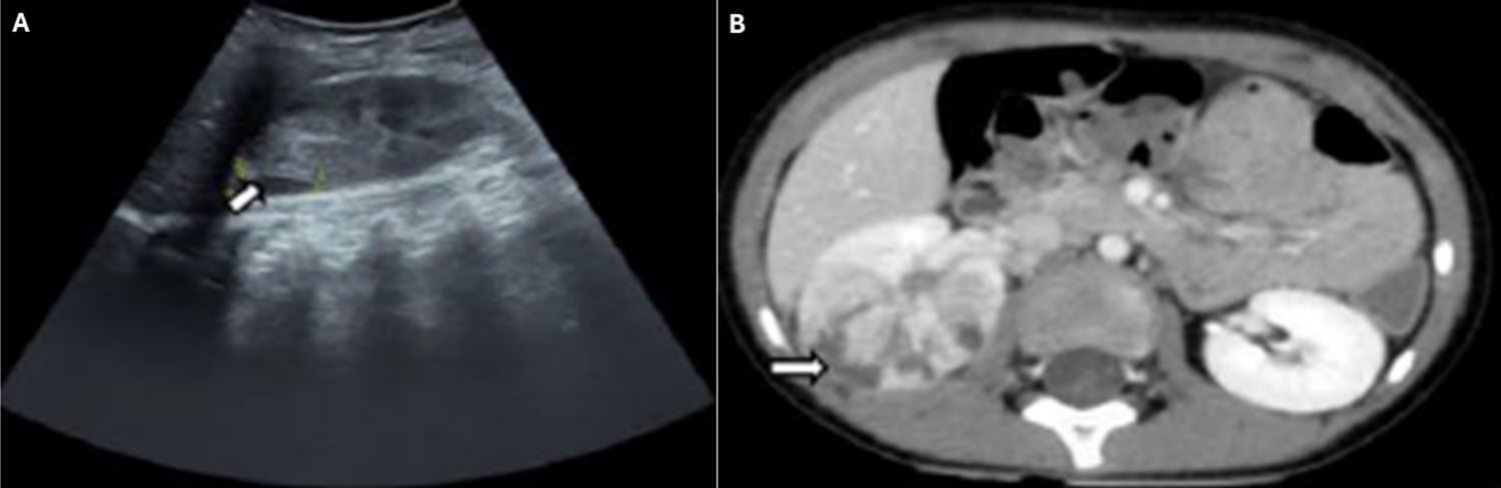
Radiological images of kidney abscess. A US reveals a well-defined hypoechoic lesion (arrow). B Enhanced CT scan displays a well-defined, low-density mass in the upper right kidney, suggestive of a kidney abscess (arrow). Adapted from reference [31], reproduced under Creative Commons License CC BY. Ultrasound, US; computed tomography, CT
